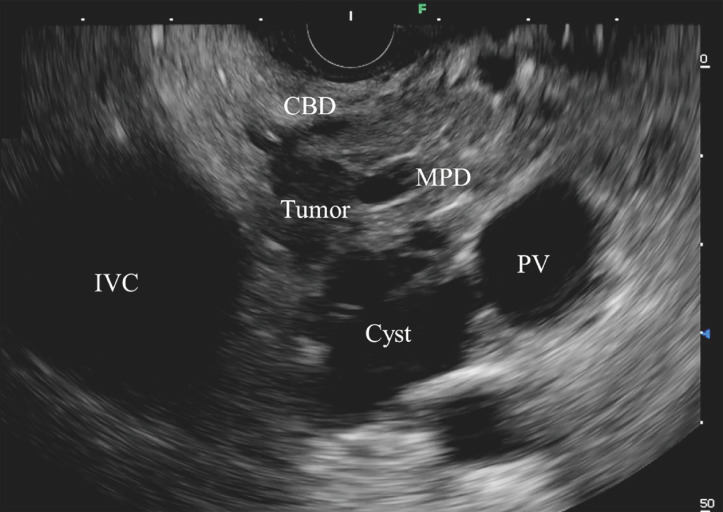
Endoscopic ultrasound shows a hypoechoic tumor adjacent to the multilocular cyst.
